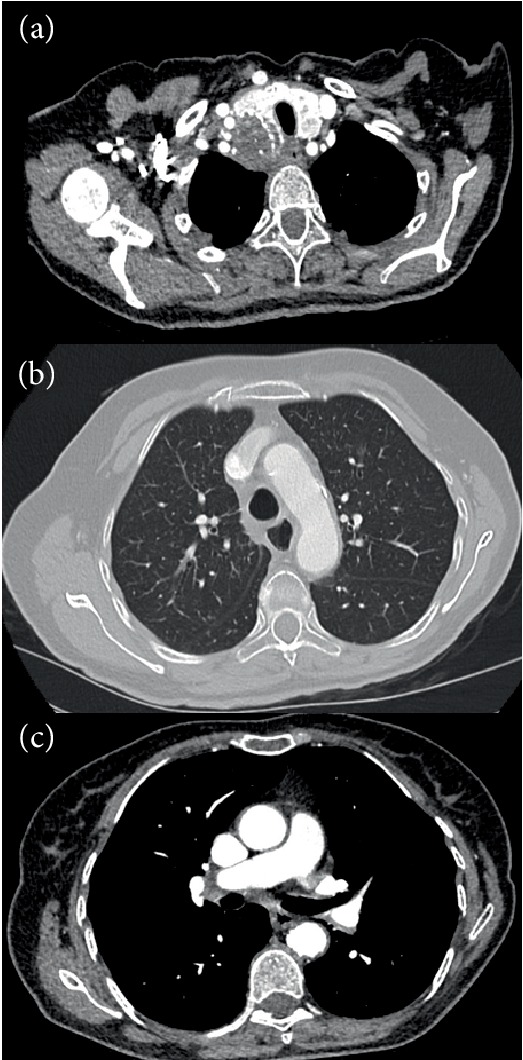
Chest CT after 3 cycles of pembrolizumab showing marked regression of primary tumor (a), lung metastases and (b), lymph nodes (c).
